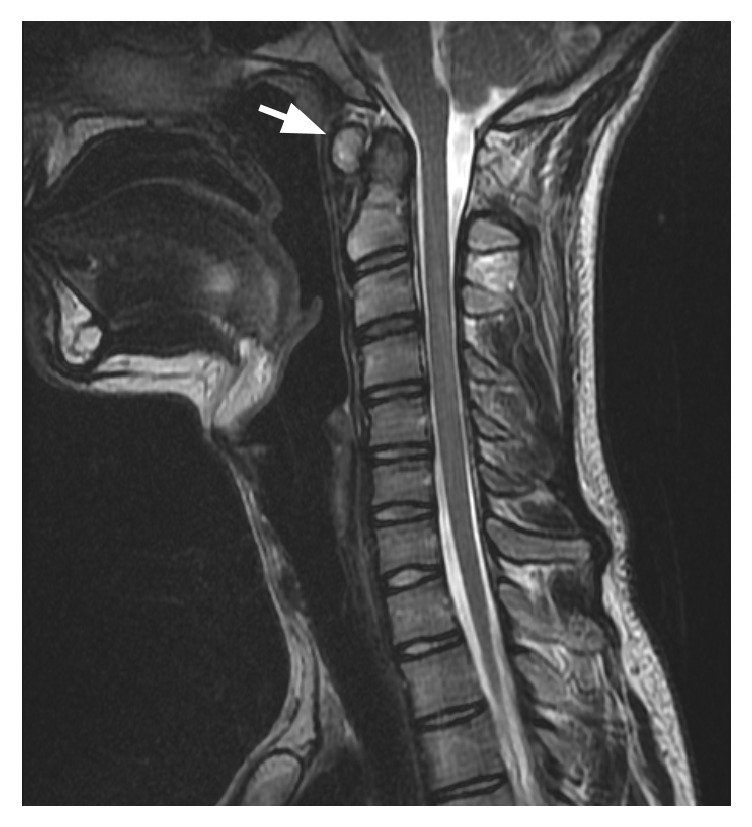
Cervical MRI image revealing slight dysplastic enlargement of the anterior arch of C1 vertebrae (white arrow).
